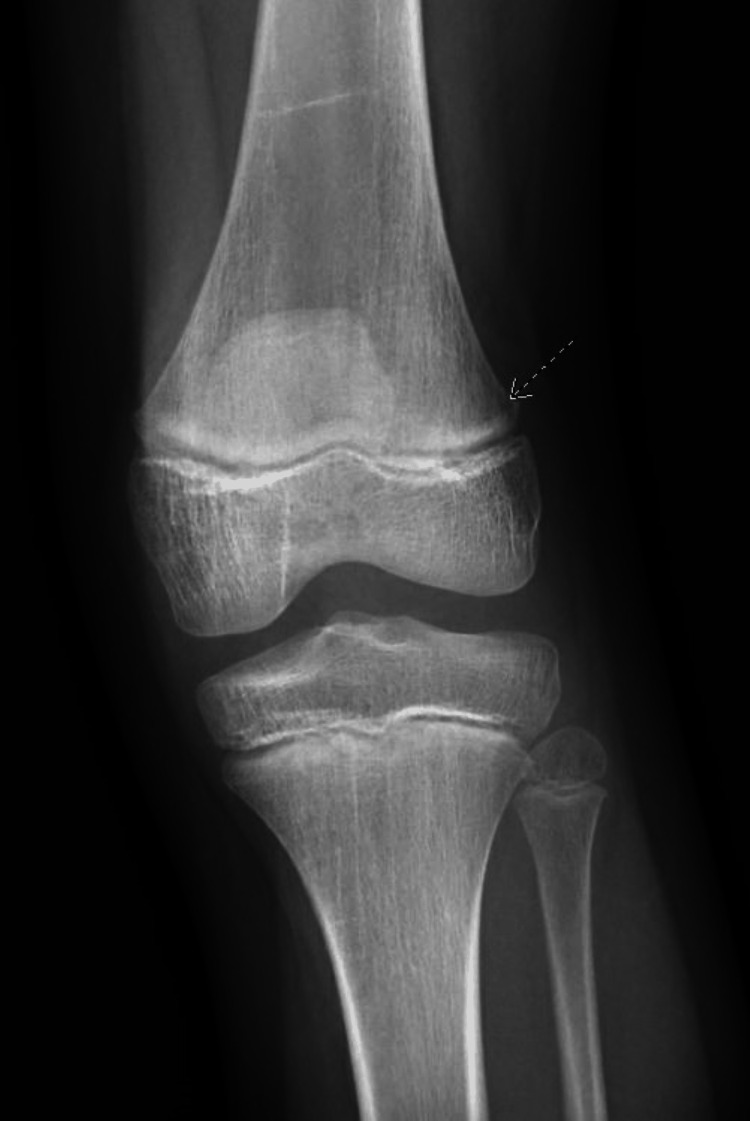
X-ray imaging showing a lytic lesion found on the distal metaphysis of the left femur (arrow).
